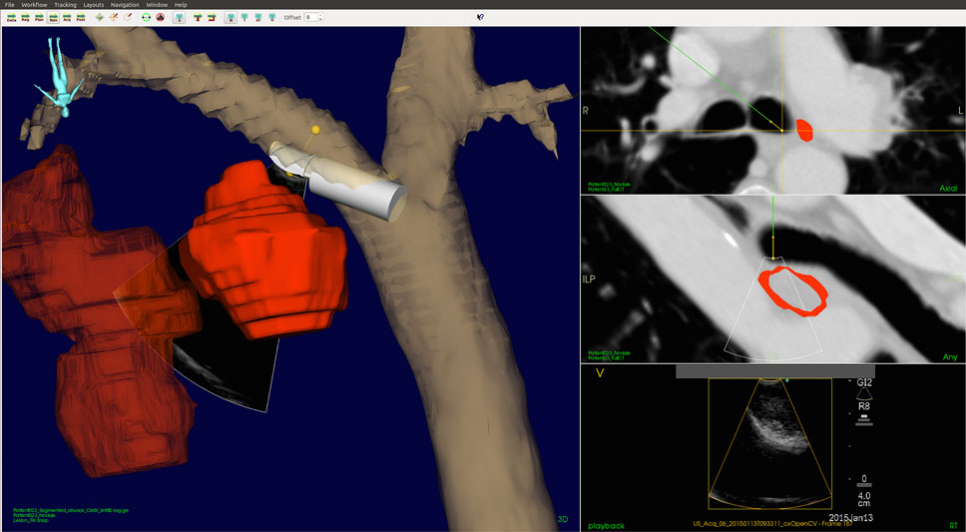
A navigation scene during EBUS-TBNA. *Left* 3D representation of the segmented airways, EBUS scope, lymph node, and tumor. *Right* Axial view of CT (*top*), view of CT corresponding to the US sector (*middle*), and US (*bottom*)
